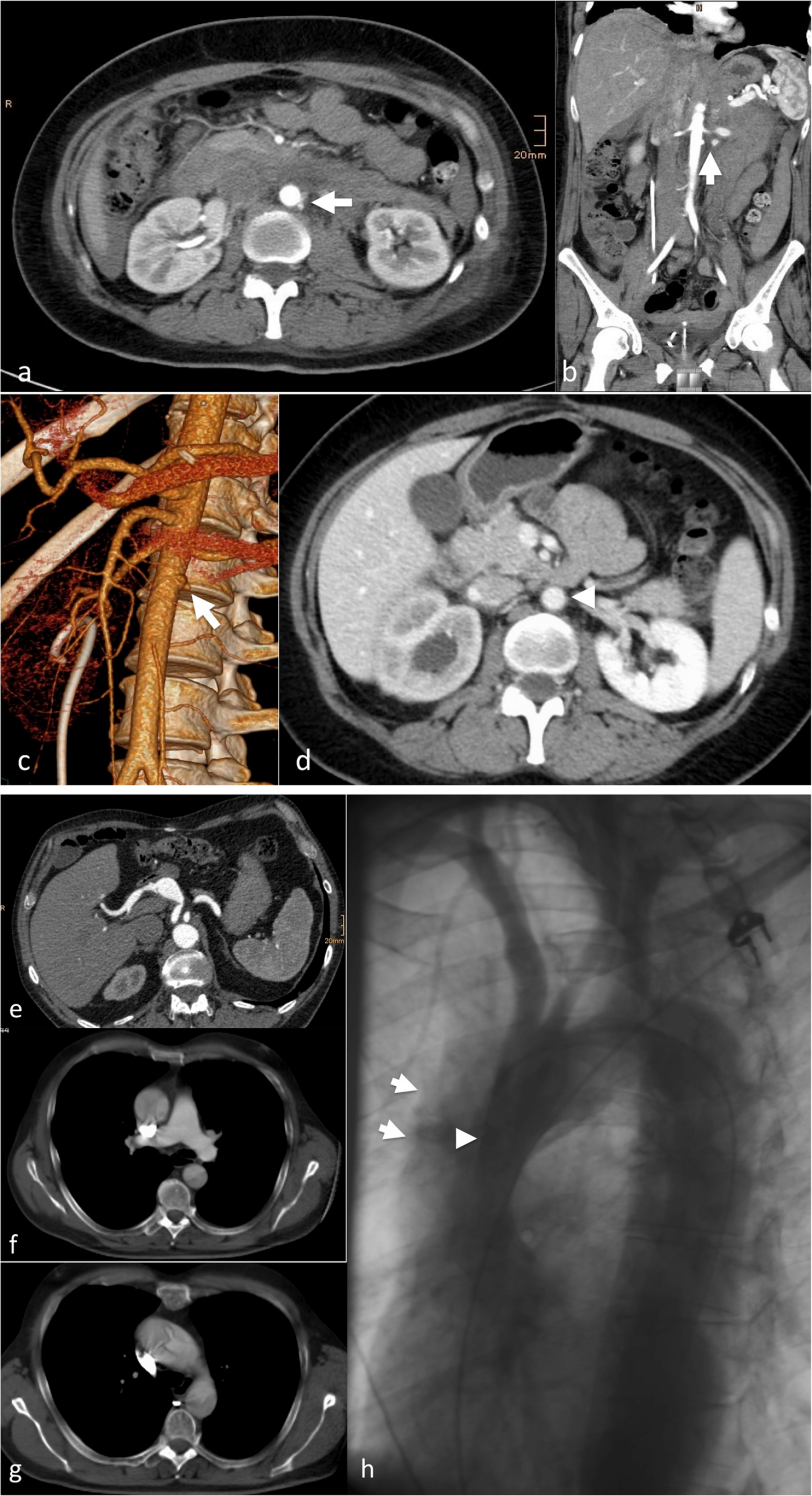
Iatrogenic aortic injury. A 36-year-old female patient underwent laparoscopic removal of an ovarian cyst; 6 h later she presented with hypotension and severe abdominal pain. CTA [(a) axial section, arrowhead; (b) coronal reformation; (c) volume-rendered image, *arrow*] demonstrated a left lateral laceration of the aorta, confirmed to be a trocar injury during vascular surgical reconstruction. A 6-month follow-up CT demonstrates a rather smooth aortic contour at the level of the original injury (d
